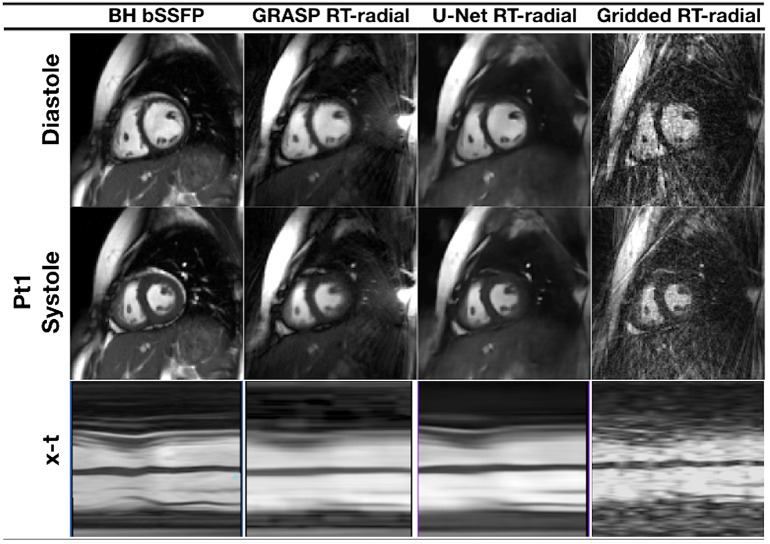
Cine MRI images for one representative patient with congenital heart disease, acquired with prospective undersampling of 13-fold. Reconstructed images are presented in peak systole and peak diastole for a reference breath-held balanced steady-state free precession sequence (BH-bSSFP, first column), the real-time radial sequence reconstructed with GRASP (82) (second column) and the residual 3D U-net (third column), as proposed in Ronneberger et al. (67). Images reconstructed with GRASP and the proposed residual 3D U-Net show spatial and temporal blurring, that could be a result of undersampling and incomplete motion correction.
